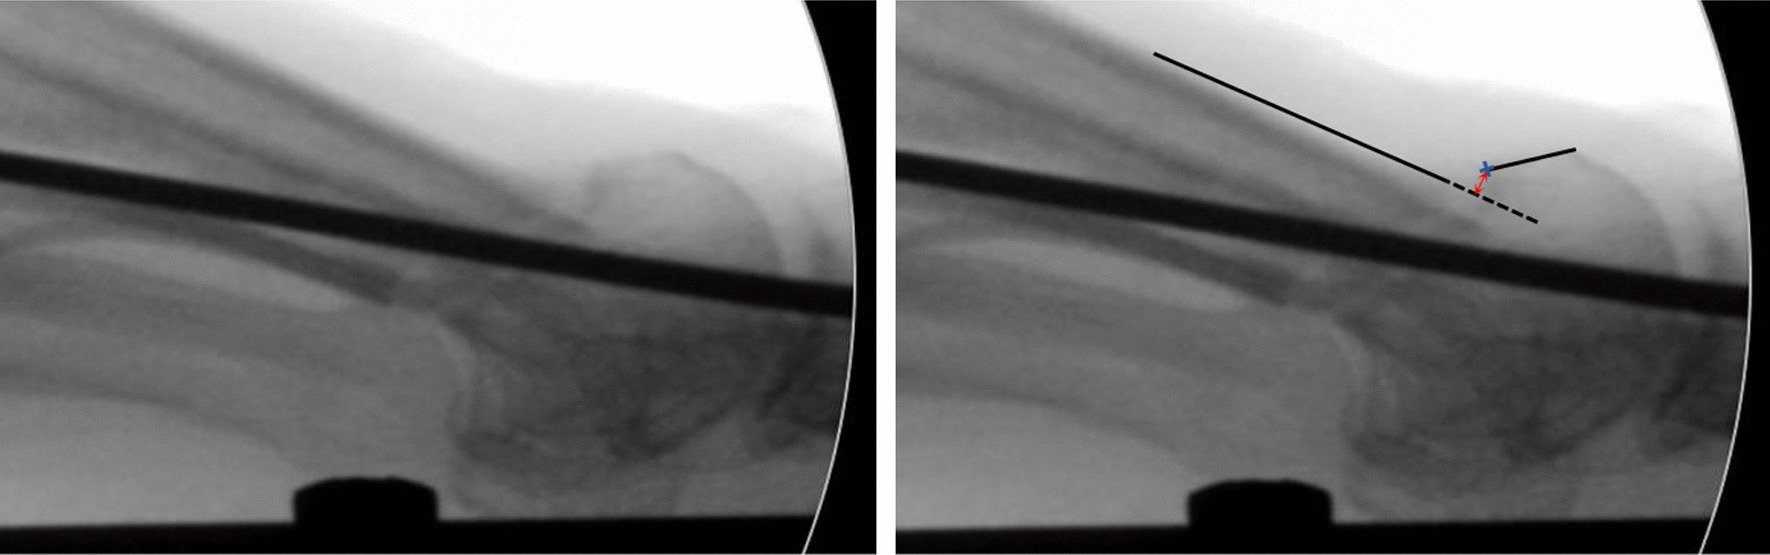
Method used to measure fragment displacement on lateral X-ray. On the left, dorsal displacement of the metatarsal head can be observed in a lateral X-ray of a Bösch osteotomy. On the right, the same X-ray with the displacement measurement. A line is drawn following the metatarsal diaphysis (continuous black line), which continues distal to the osteotomy (dashed line). A line perpendicular to the diaphyseal line is drawn (red line) from the start of the metaphyseal fragment (blue cross). This measures the distance between fragments
